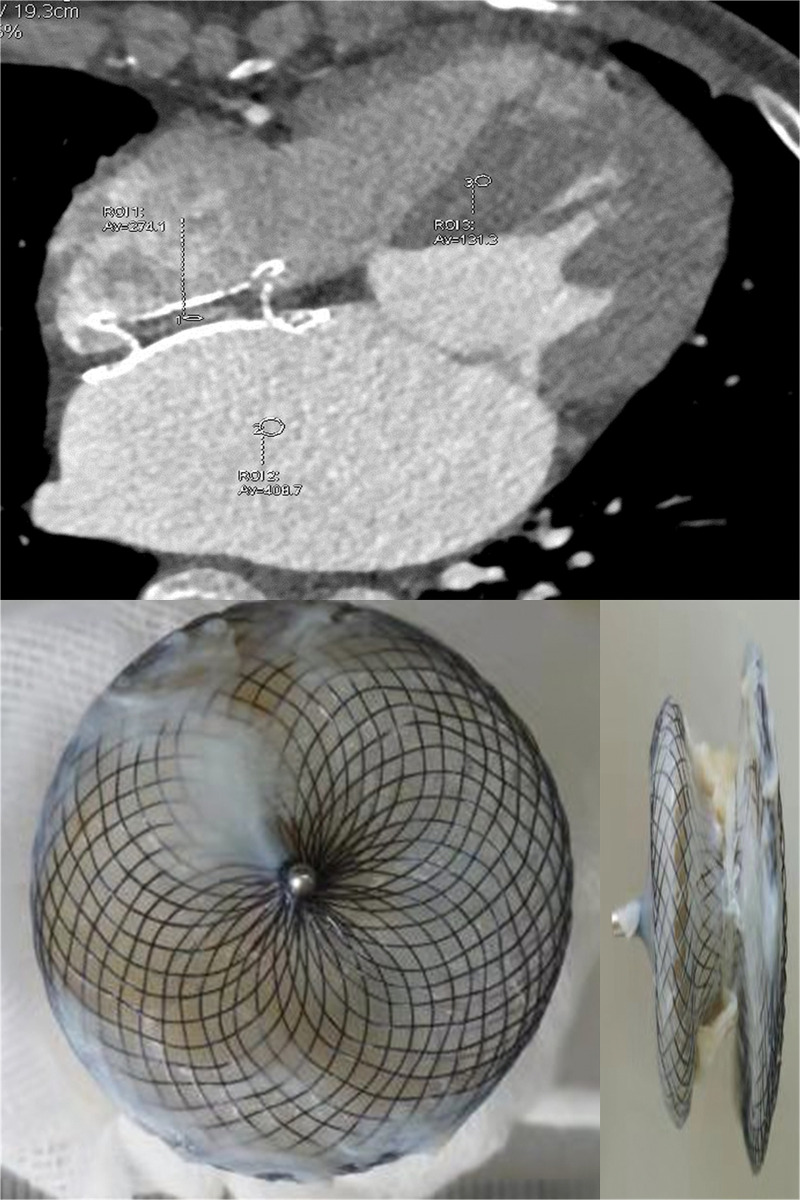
Partial contrast uptake within the occluder on cardiac computed tomography angiography (CTA). Partial contrast uptake was identified within the occluder in a patient with an implantation period of 2 years (upper panel). The local CT density of the contrast uptake area within the device exceeded that of the myocardium, and partial endothelialization was detected on the disc of the device (lower panel). The tissue from the central area (arrows) on the device indicated fibrotic tissue on the partially endothelialized device. *Note*: *local CT density was 274*.*1 HU within the contrast uptake area of the device*, *131*.*3 HU in the myocardium*, *and 408*.*7 HU in the left atrium; ROI*, *region of interest; Av*, *average CT density; CT*, *computed tomography; HU*, *Hounsfield units*.
